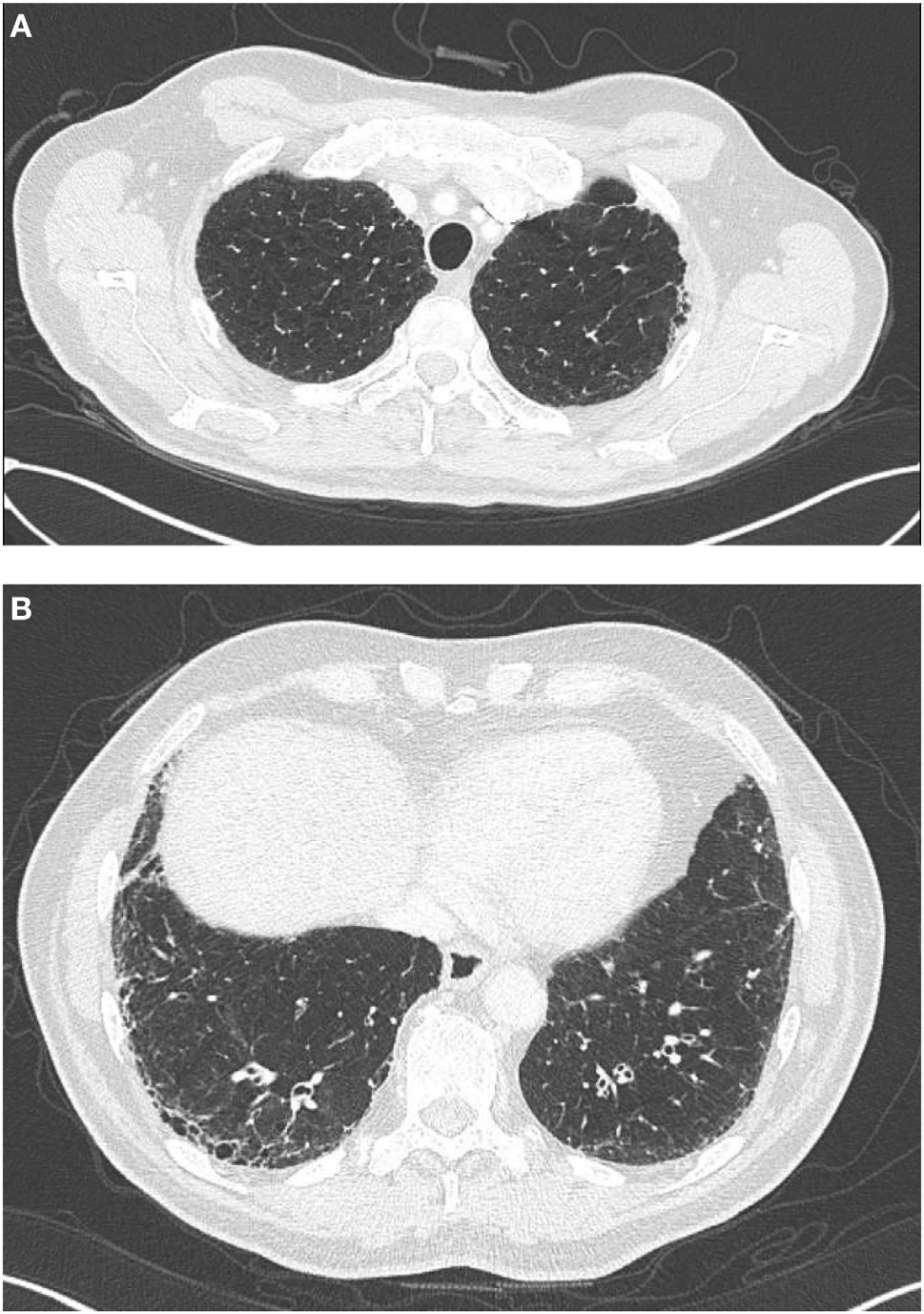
High-resolution computed tomography findings in a patient, with combined pulmonary fibrosis and emphysema. Centrilobular emphysema is observed on apical views (A) and basilar predominant sub-pleural reticulation and honeycombing characteristic of UIP is observed on basilar views (B).
